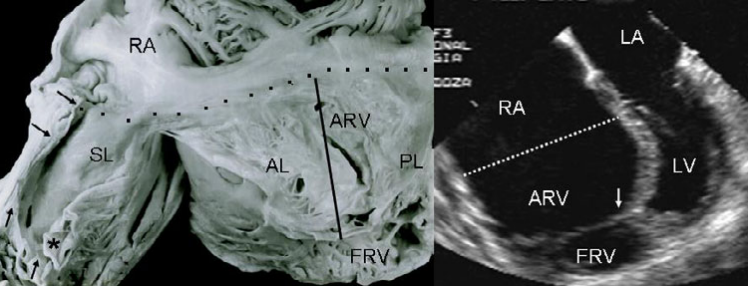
Internal view of the right chambers of a heart shows grade III tethering of the tricuspid septal leaflet. The arrows point to tethering of the posterior leaflet. The flap of the free portion of the septal leaflet can be seen (asterisk) as well as the redundant anterior leaflet with 3 accessory openings. The 4 chamber echocardiographic image shows the same features and the greater size of the atrialized portion of the right ventricle with significant reduction of the functional portion. Abbreviations as before.
